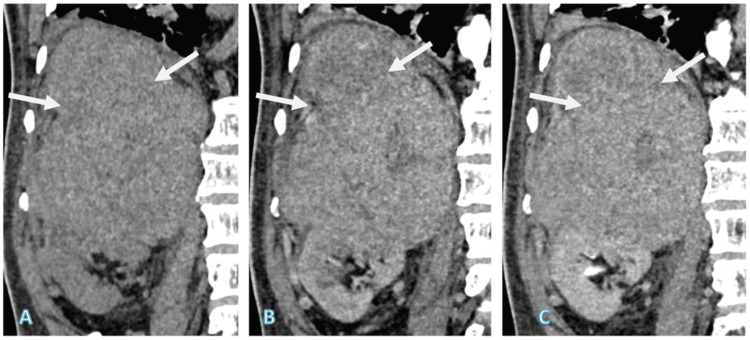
Adrenocortical carcinoma: coronal CECT shows a large heterogeneous right adrenal mass (white arrows) with high unenhanced attenuation (A), irregular margins, necrosis, mild non-uniform enhancement (B) and suboptimal washout (C), consistent with adrenocortical carcinoma. CECT = contrast-enhanced CT
